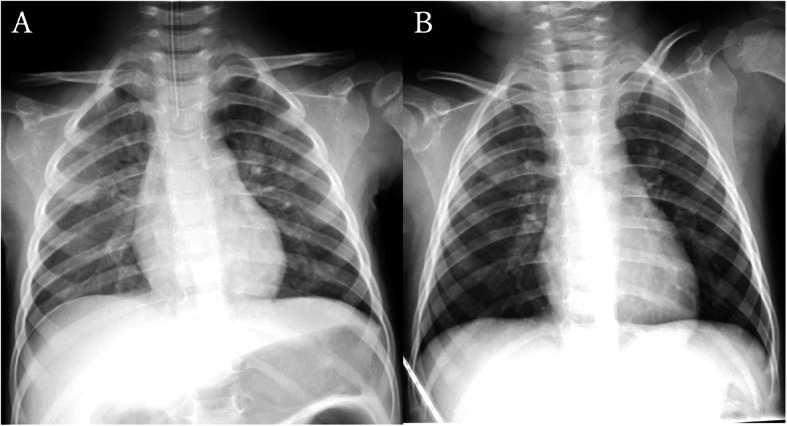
Chest radiograph findings. a. Revealing bilateral, centrally distributed opacities, without cardiac dilation upon ER arrival. b. Remarkable improvement of lung consolidation can be observed on hospital day 2
